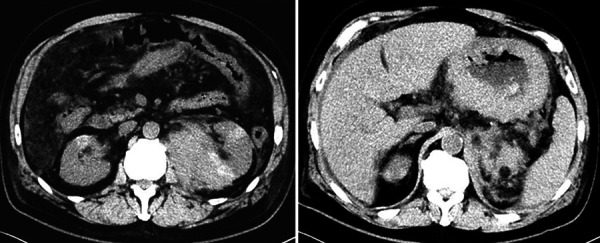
Abdominal CT scan with contrast. Findings of bilateral enhancing lesions in the kidneys. Multiple rounded hyperdense lesions throughout the renal parenchyma bilaterally, more pronounced in the left kidney. Left perinephric hematoma 8.8 × 5.2 cm. Axial view (left). Diffuse thickening of the stomach wall. No evidence of liver lesions. Axial view (right).
